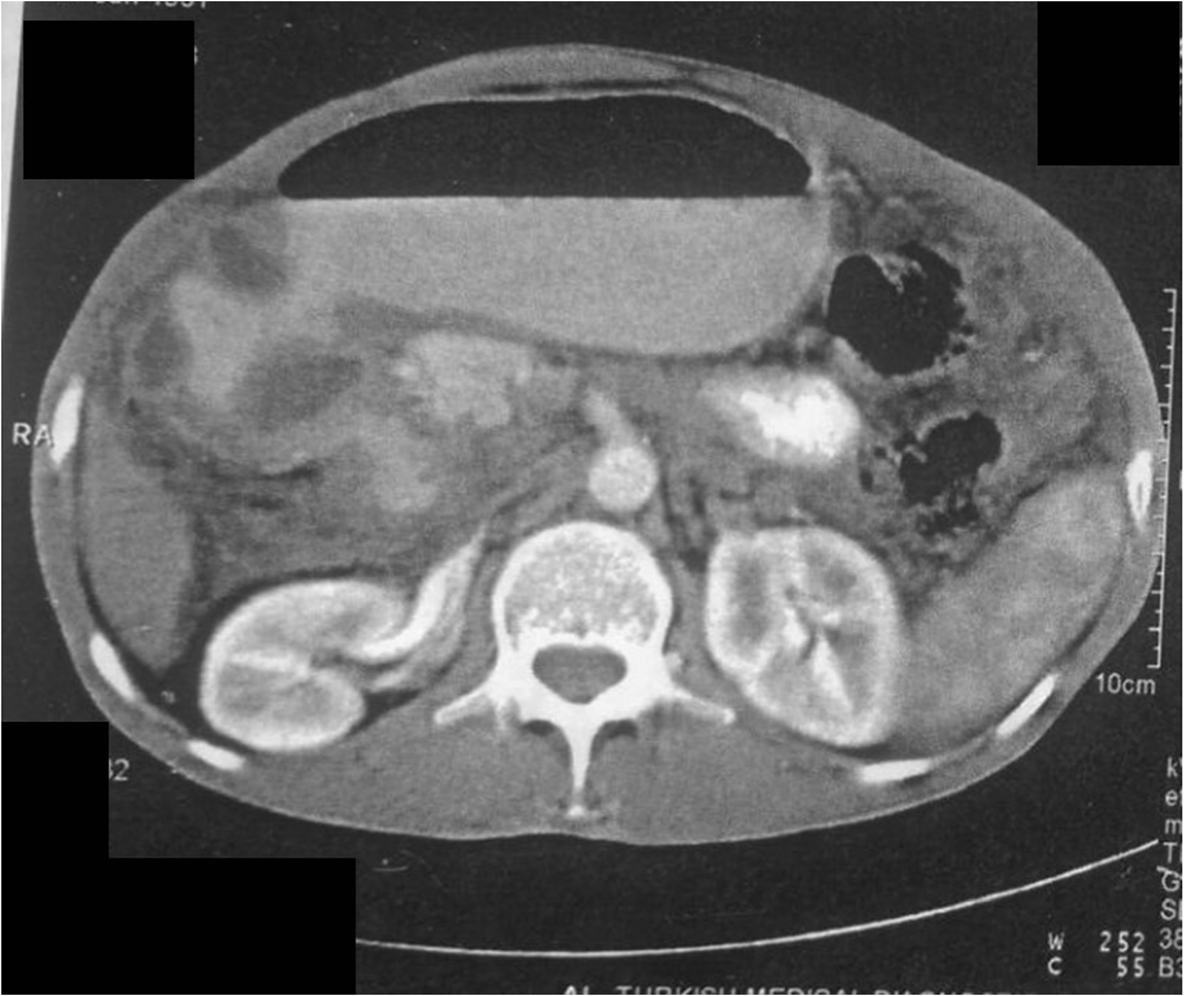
A computed tomography scan of the abdomen showed nodular hypodense lesions measuring 30 mm surrounding the antrum of the stomach with gastric dilatation, and multiple mesenteric lymphadenopathies measuring 40 mm
